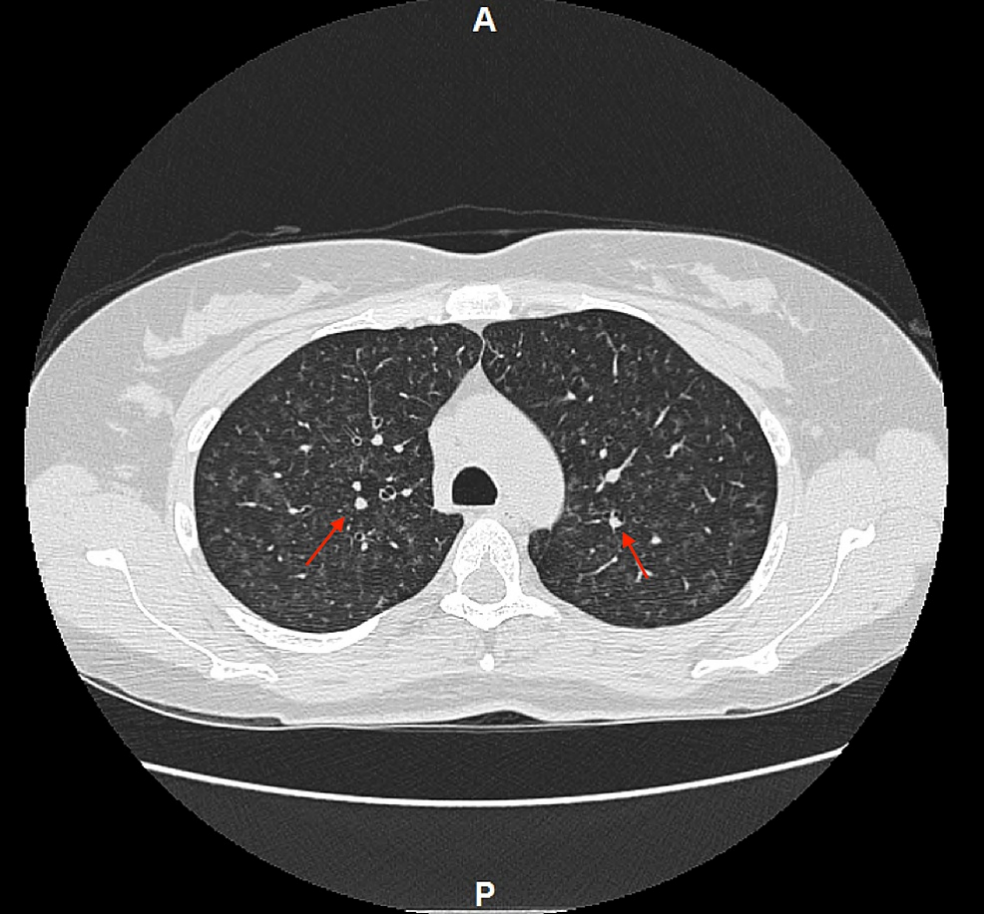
Chest CT showing innumerable pulmonary micronodules in the lungs bilaterally most prominent in the mid and upper lung zones with patchy ground-glass opacity and tree-in-bud opacities
